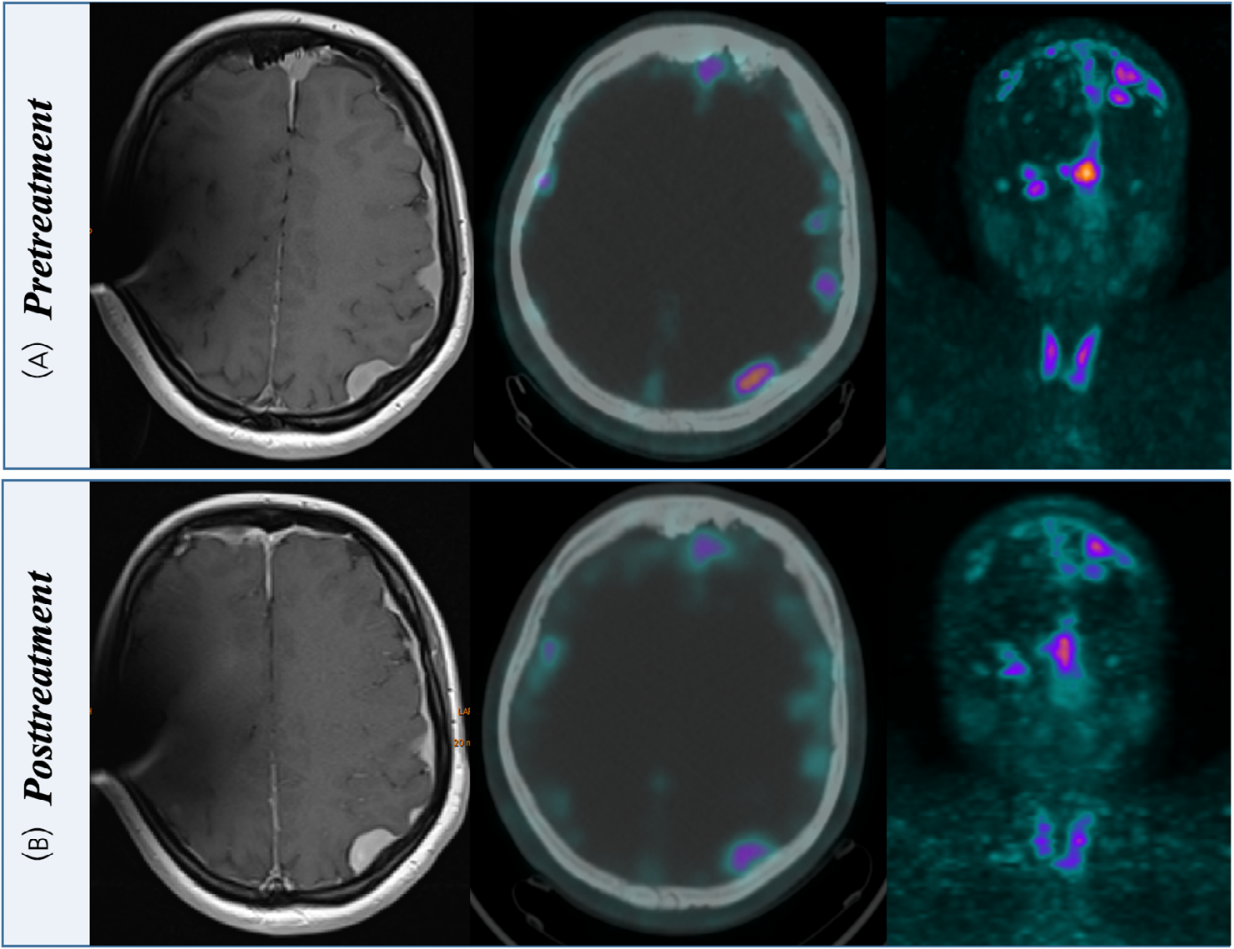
A 29‐year‐old patient suffering from neurofibromatosis Type II with multiple meningiomas and vestibular schwannoma. The patient underwent four cycles of ^177^Lu‐DOTATATE with a total administered activity of 29.97 GBq until April 2023. Pre‐treatment MRI and ^68^Ga‐DOTATOC PET/CT (A) showed multiple meningiomas, mostly supratentorial. Post‐treatment images (B) showed a minor response to PRRT. Fourteen months after the last course of therapy, the patient is still stable on imaging evaluation. PET, positron emission tomography; PRRT, peptide receptor radionuclide therapy.
